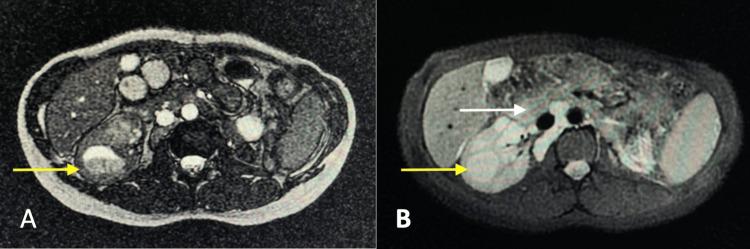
(A) Balanced turbo field echo (BTFE) MRI sequence showing a left renal mass with heterogeneous signal intensity (yellow arrow). (B) Diffusion-weighted MRI (b-0) demonstrating the same renal mass (yellow arrow) along with enlarged retroperitoneal lymph nodes (white arrow), suggestive of disease progression from renal cell carcinoma.
